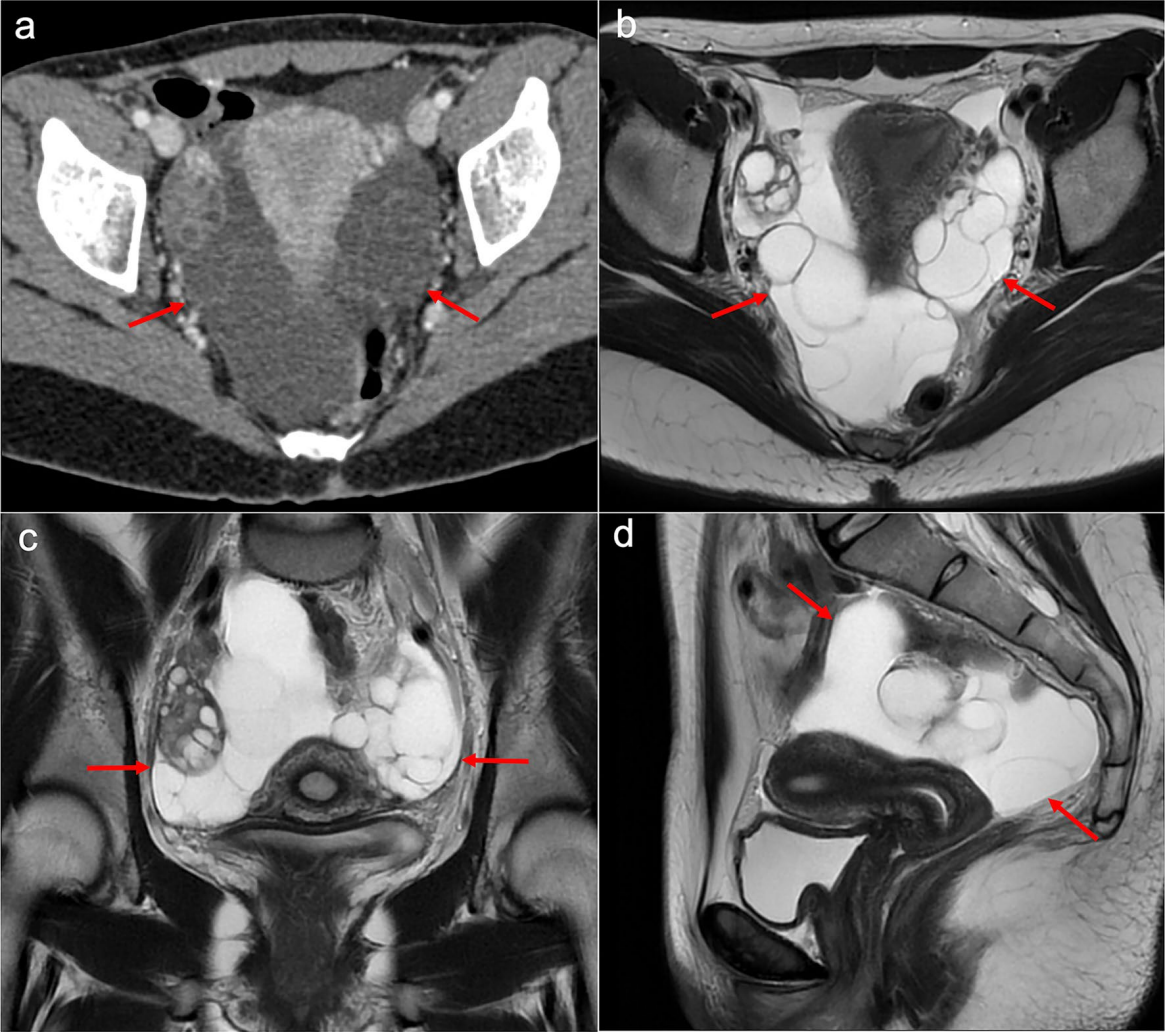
Benign multicystic peritoneal mesothelioma in a 21-year-old woman with abdominal pain. a Axial CT image in the portal phase shows a hypodense multilocular pelvic mass of cystic appearance with thin septa (red arrows). T2-weighted MR images of the pelvis in axial (b), coronal (c) and sagittal (d) planes confirm the presence of a hyperintense multilocular cystic pelvic mass surrounding the uterus and ovaries (red arrows), containing clusters of thin-walled cysts with a grapelike morphology. The suspected diagnosis of a benign multicystic peritoneal mesothelioma was confirmed after laparoscopic surgery
